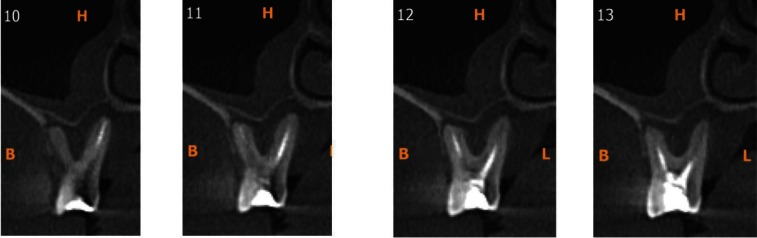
Para-axial section showing the periapical condition.
